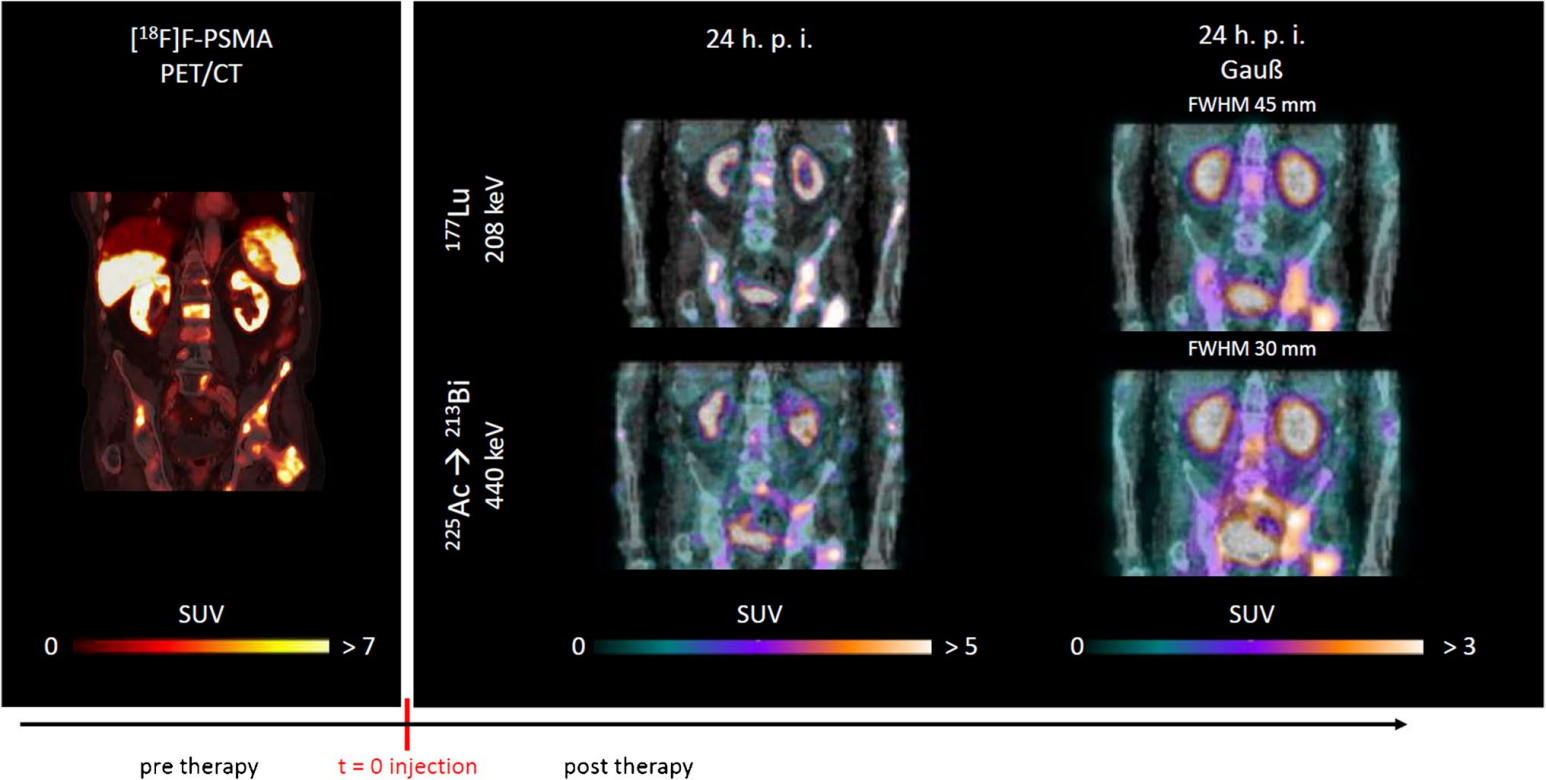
Coronal slices for patient 5; the pre-therapeutic PSMA PET/CT is shown together with the filtered and unfiltered therapeutic quantitative SPECT/CT for [^177^Lu]Lu-PSMA-I&T and [^225^Ac]Ac-PSMA-I&T at 24 h p. i
